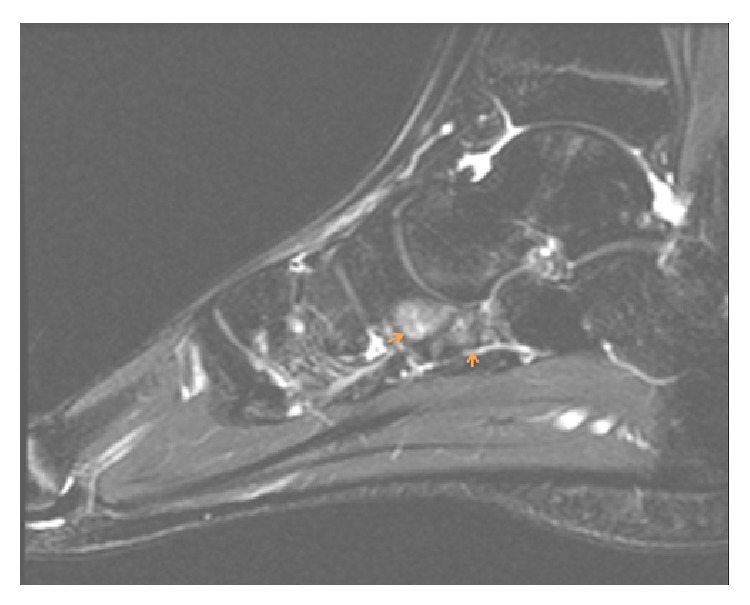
Sagittal STIR unenhanced MR image of the right foot in a 17-year-old male with cuboid-navicular coalition reveals abnormal articulation between the cuboid and navicular as well as marrow edema along both sides of the coalition (arrows).
